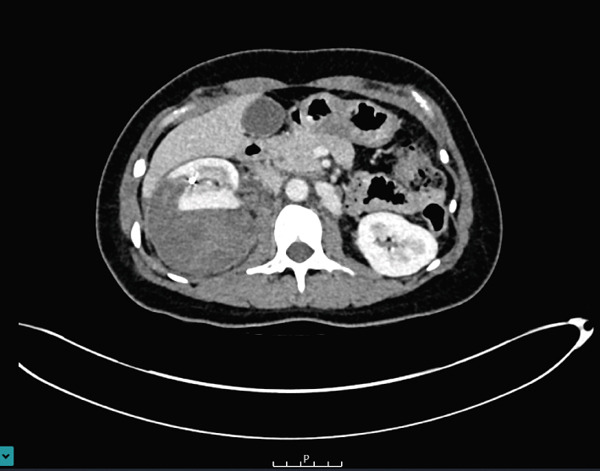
Axial CT image demonstrated a stable in size perinephric haematoma extending to the right psoas muscle.
